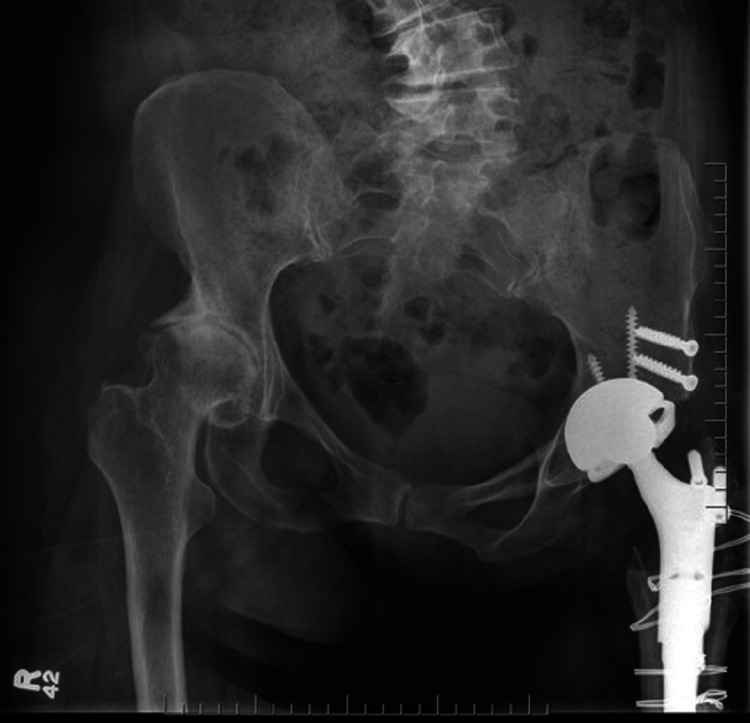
Post-operative image Post-operative anteroposterior radiograph. Conversion of left side hip fusion to THA was successfully completed.
